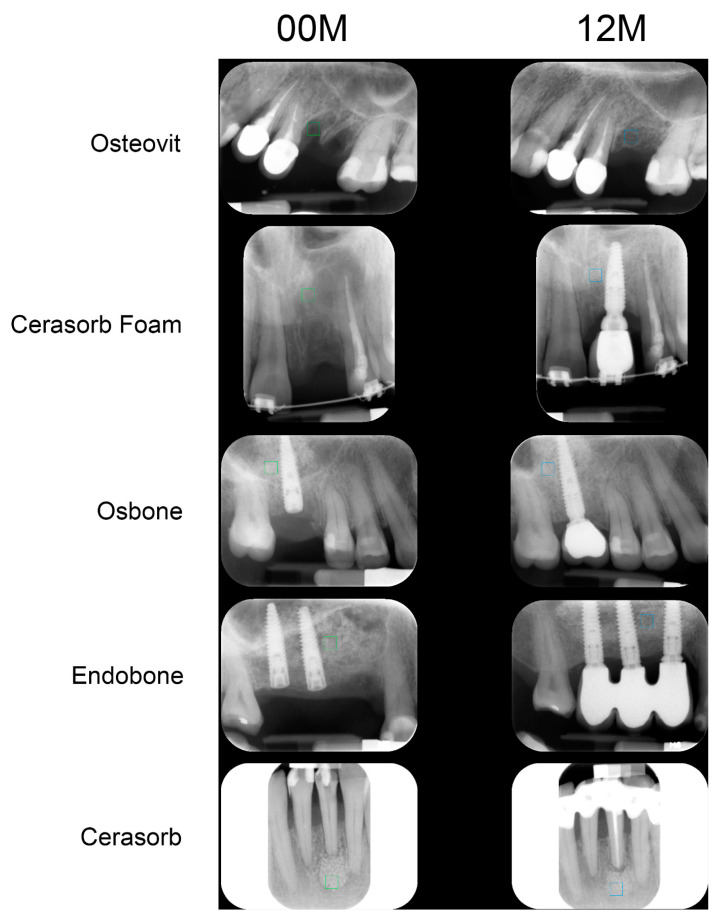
Examples of analyzed intra-oral radiographs (directly after implantation—00M, one year after surgery—12M). The exemplary regions of interest are marked in green and blue.
